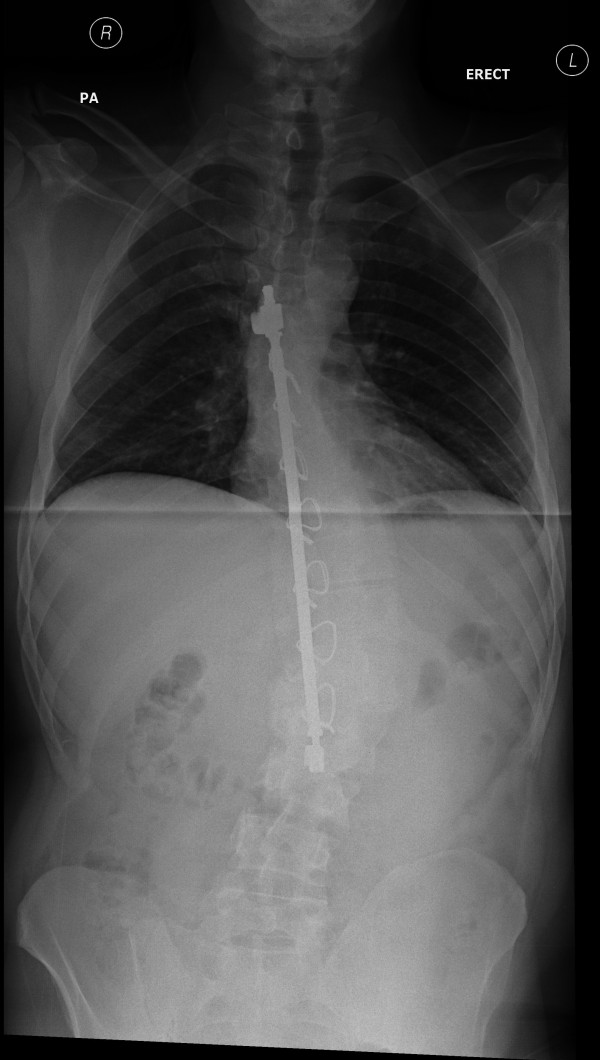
A pre-operative whole-spine standing radiograph image of the Harrington rod and the scoliosis 22 years after implantation at the index surgery.
